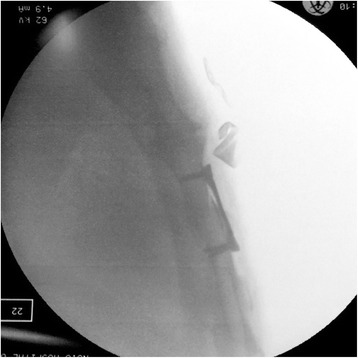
An intraoperative, portable wet-film radiography image of the sternum
